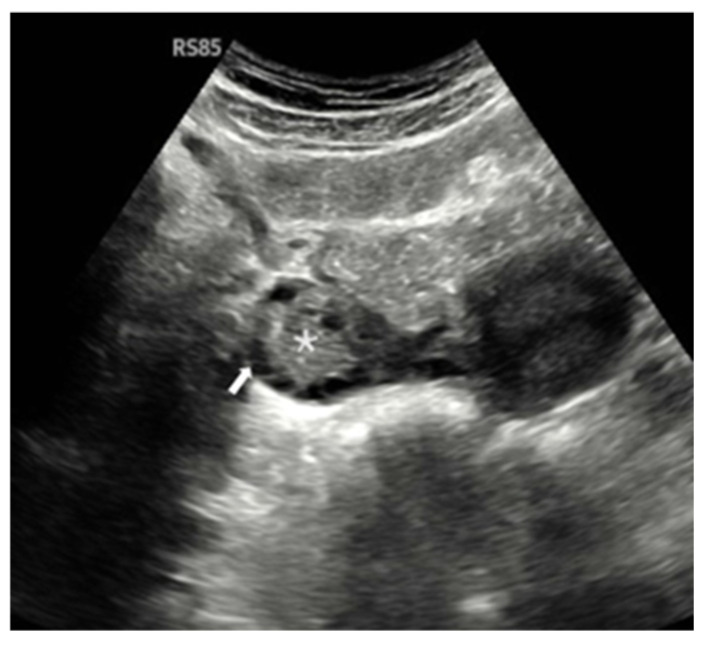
Gray-scale transabdominal ultrasound image in a 22-year-old female demonstrating polycystic ovarian morphology (PCOM). The central ovarian stroma is marked by an asterisk (*), and multiple peripheral follicles (each measuring 2–9 mm in diameter) are indicated by the white arrow. The ovarian volume was calculated as 12 mL. The presence of a prominent central stroma, numerous peripherally arranged follicles, and increased ovarian volume are consistent with PCOM.
